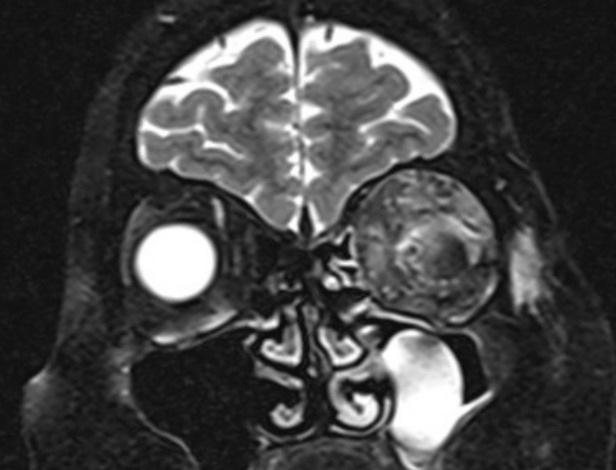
MRI scan, coronal view showing left extraocular muscle enlargement along with the enlargement of lacrimal gland
